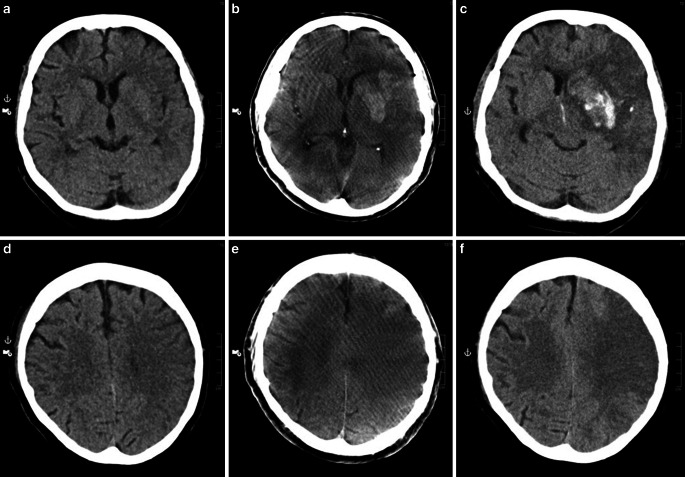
Imaging over time in a case of acute carotid‑T occlusion. a/d show qualifying image (multi-slice CT) for endovascular therapy with preserved contrast of basal ganglia (a) and frontal and parietal lobes (d). b/e show contrast extravasation in basal ganglia (b) and beginning demarcation in the frontal and parietal lobes (e), (sinusoidal flat-panal CT after endovascular therapy). c/f show 24 h follow-up (multi-slice CT) with progressive demarcation of the basal ganglia (c) and more apical levels (f)
